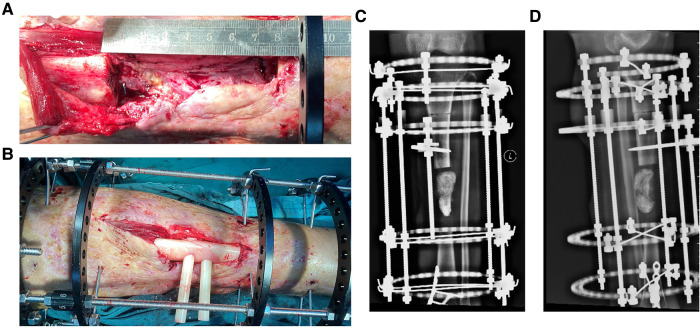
The osteotomy was carried and intraoperative application of PSI in Ilizarov technique of distraction osteogenesis and the postoperative x-ray image in case 2. (A) Intraoperative image of case 2 after osteotomy. (B) The PSI was put into the bone defect to assist reduction. (C) x-ray image on Anterior-posterior (AP) view after operation of case 2, and the iodoform gauze were filled in bone defect. (D) x-ray image on lateral view after operation of case 2.
